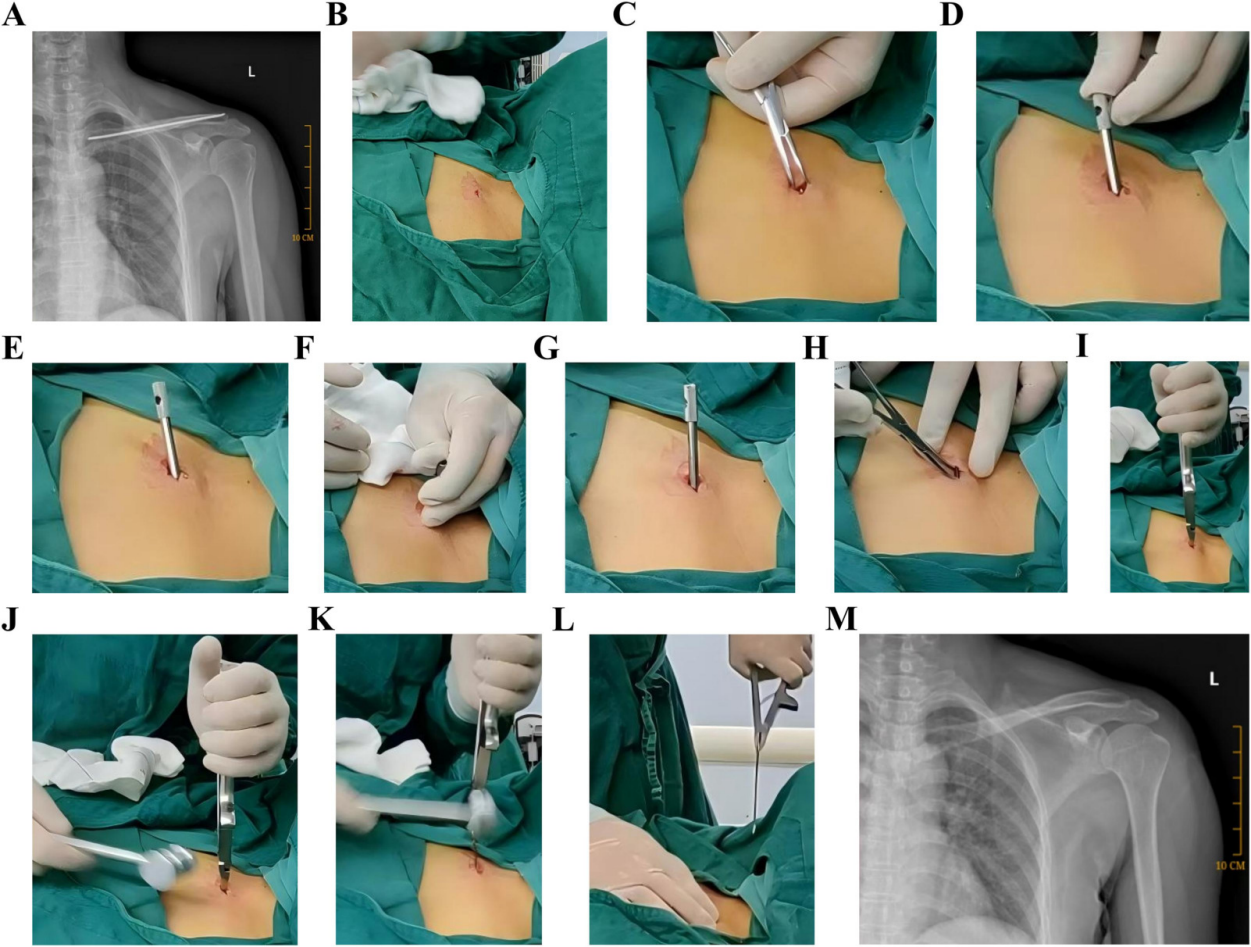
K-wire sleeve-assisted ESIN removal from an adult clavicle. (A) Postoperative radiograph confirming fracture union (1-year follow-up); (B) original incision reopening to expose ESIN terminus; (C) hemostat-assisted exposure expansion; (D) Sleeve insertion along the ESIN terminus without incision enlargement; (E) initial angulation (30°) of the ESIN terminus; (F) controlled bending via the sleeve; (G,H). Final angulation (70–90°); near-orthogonal configuration); (I) perpendicular engagement of clamping instruments; (J–M) mallet-assisted ESIN extraction. ESIN, elastic intramedullary nails; K-wire, Kirschner Wire.
